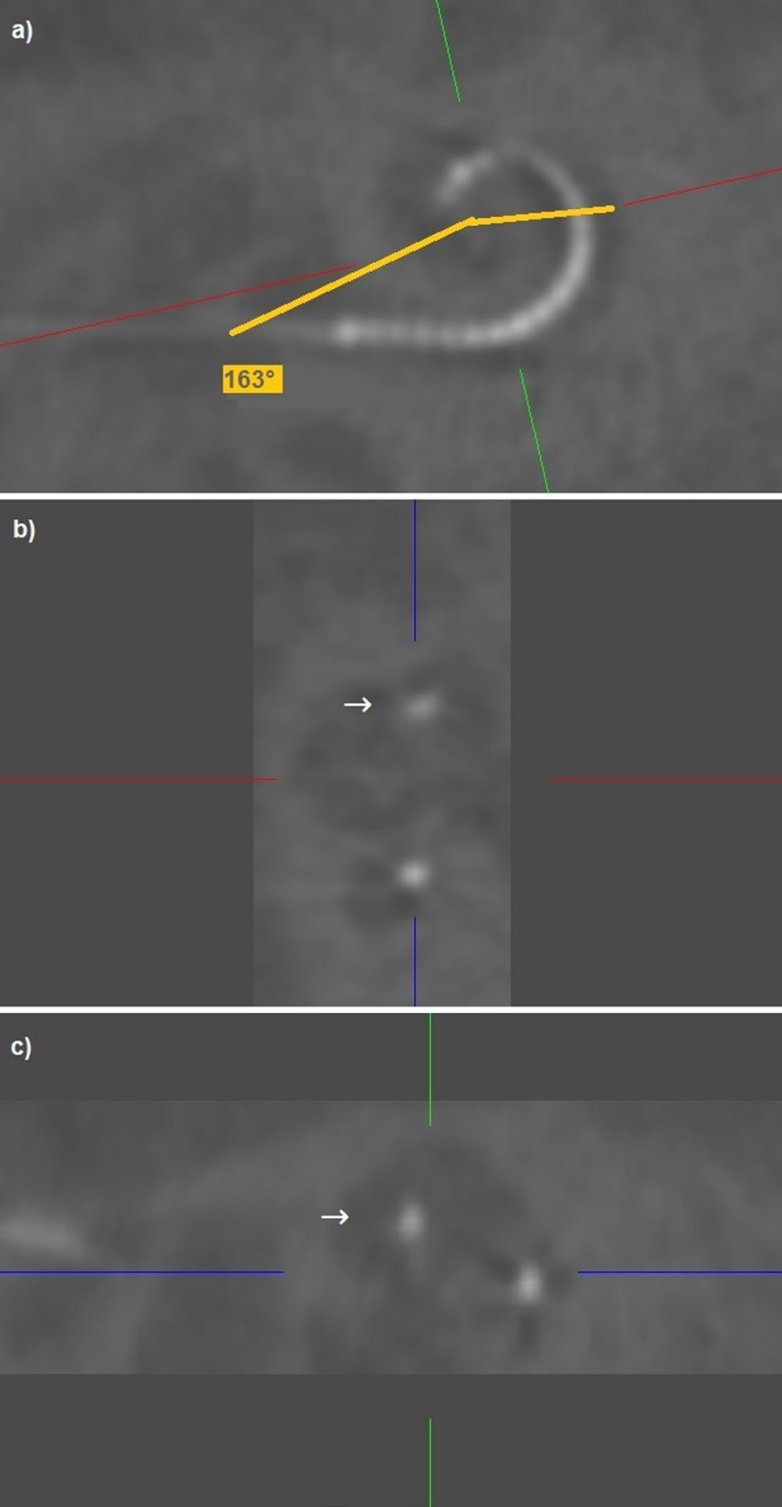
Three-dimensionally reconstructed CBCT: the SMA inserted via round window approach dislocated at 163° (a). b and c show the array initially in ST and clearly in SV after 163° (→)
